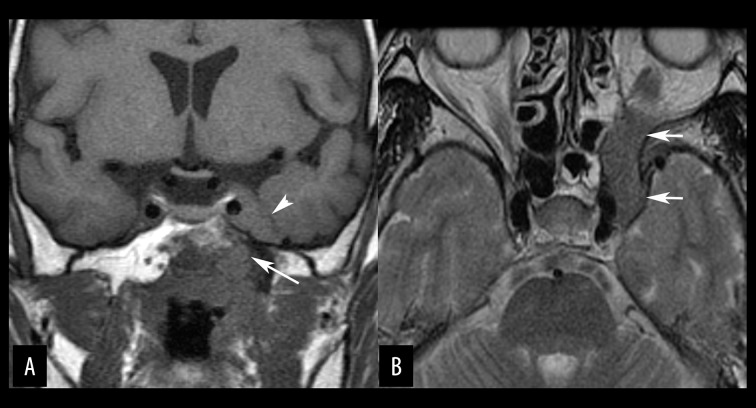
Perineural tumor spread of nasopharyngeal carcinoma in a 63-year-old woman. (A) Coronal, non-contrast, T1-weighted, MR image shows nasopharyngeal mucosal wall thickening. The tumor invades into the basisphenoid and spreads through the left Vidian canal (arrow) to the pterygopalatine fossa (not shown), and then goes up to the left cavernous sinus along the maxillary branch of the left trigeminal nerve. The left cavernous sinus is also replaced by the tumor (arrow head). (B) Axial, T2-weighted MR image shows the mass with intermediate intensity along the maxillary branch of the left trigeminal nerve (arrows).
